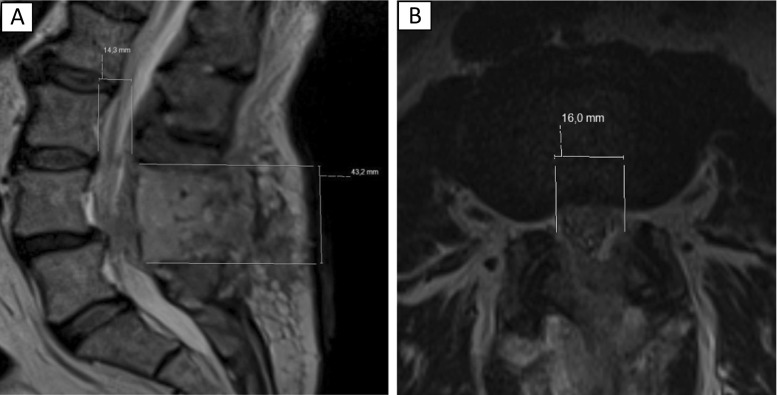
a Measurements of height (14.3 mm) and length (43.2 mm) on MRI. b Measurement of width (16.0 mm) on MRI
